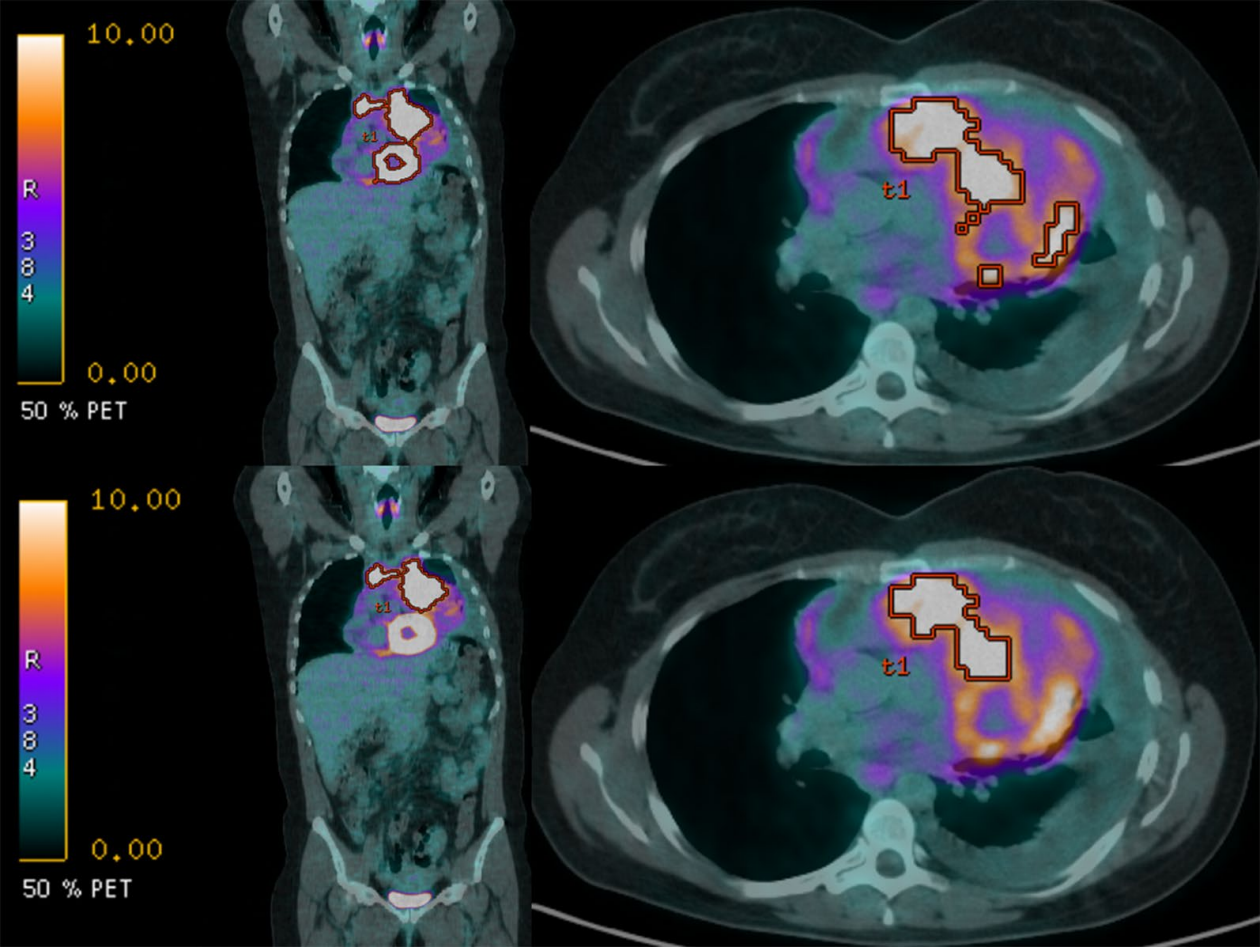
Example of an automated segmentation with manual observer verification and adjustment: Fused coronal (left) and axial (right) [^18^F] FDG PET/CT images of a mediastinal lymphoma following automated segmentation (upper), and following manual adjustment (lower). The automated segmentation used a threshold weight of 0.5 based on an estimated threshold method with the PET VCAR (General Electric) software. It includes the physiological uptake in the myocardium. Manual inspection and adjustment are required to exclude the heart
